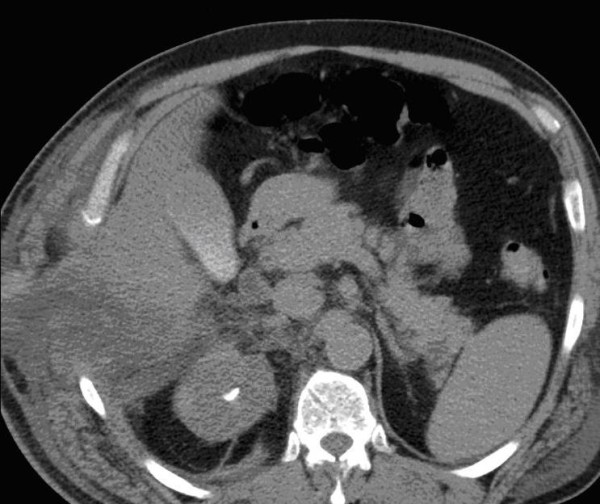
CT at 48 hours post injury: herniated segment VI of the liver without contrast enhancement, suggesting strangulation.
